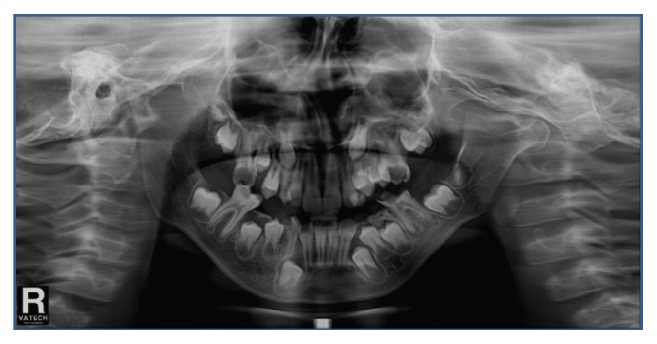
Panoramic radiograph of the patient before treatment.
